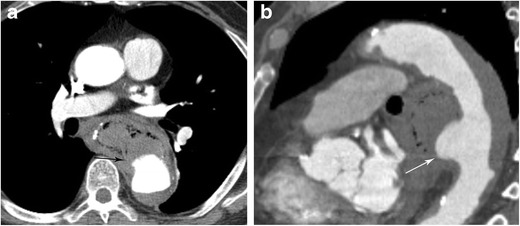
Aortoesophageal Fistula. CT angiogram of the chest in soft tissue window in axial (a) and sagittal (b) planes demonstrates extravasation of contrast from the descending thoracic aorta into the oesophagus. Soft tissue attenuation material within the oesophagus is consistent with clotted blood
